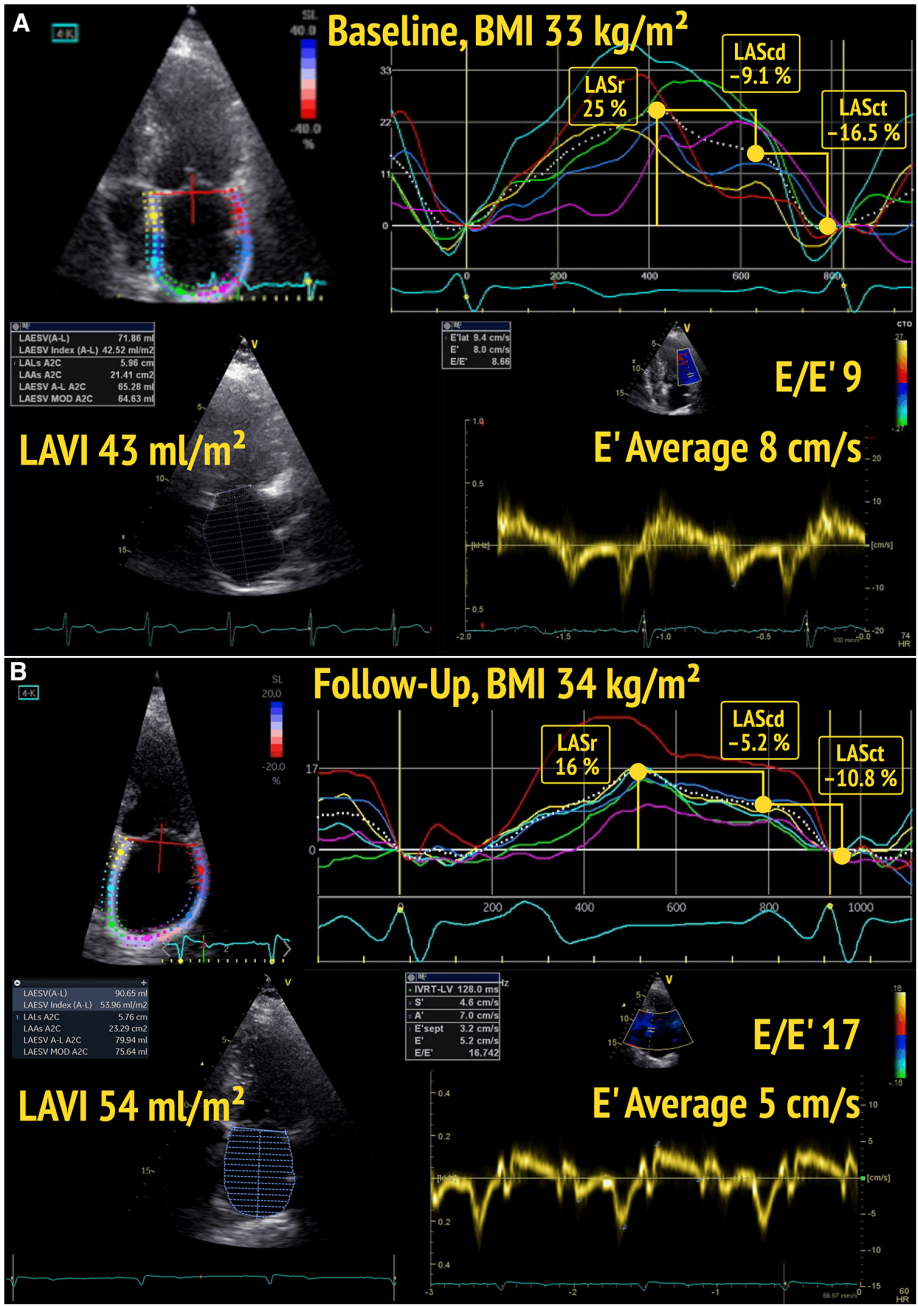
Baseline (A) and follow-up (B) echocardiography of a participant with BMI ≥25 kg/m^2^, showing a decline of left atrial reservoir strain (LASr) and left atrial conduit strain (LAScd), as well as a deterioration of diastolic function (from DD1 to DD2) over time.
